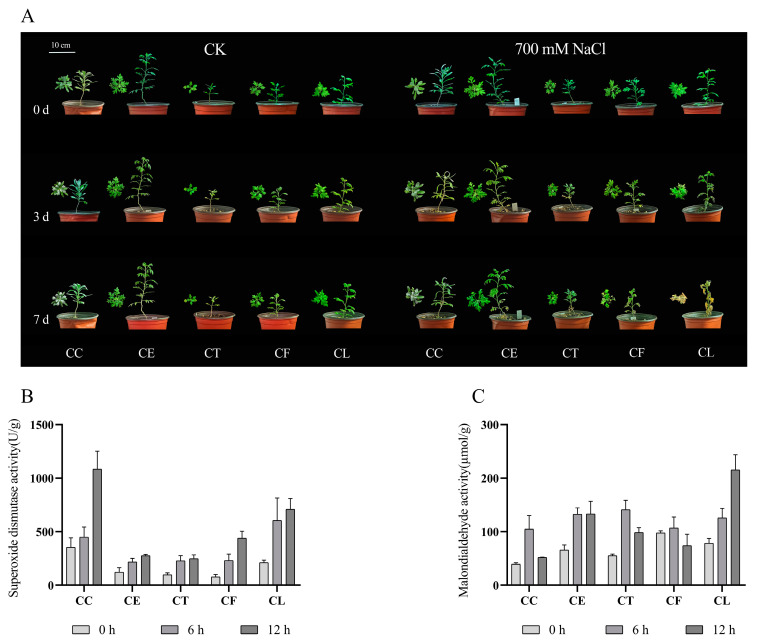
The effect of 700 mM NaCl exposure on the growth and enzymatic antioxidant activities of *Cr. chinense* (CC), *C. lavandulifolium* (CL), and three hybrids (CE, CT, and CF): (A) phenotypic differences, (B) SOD, (C) MDA. Data are means of three replicates and follow normal distribution.
